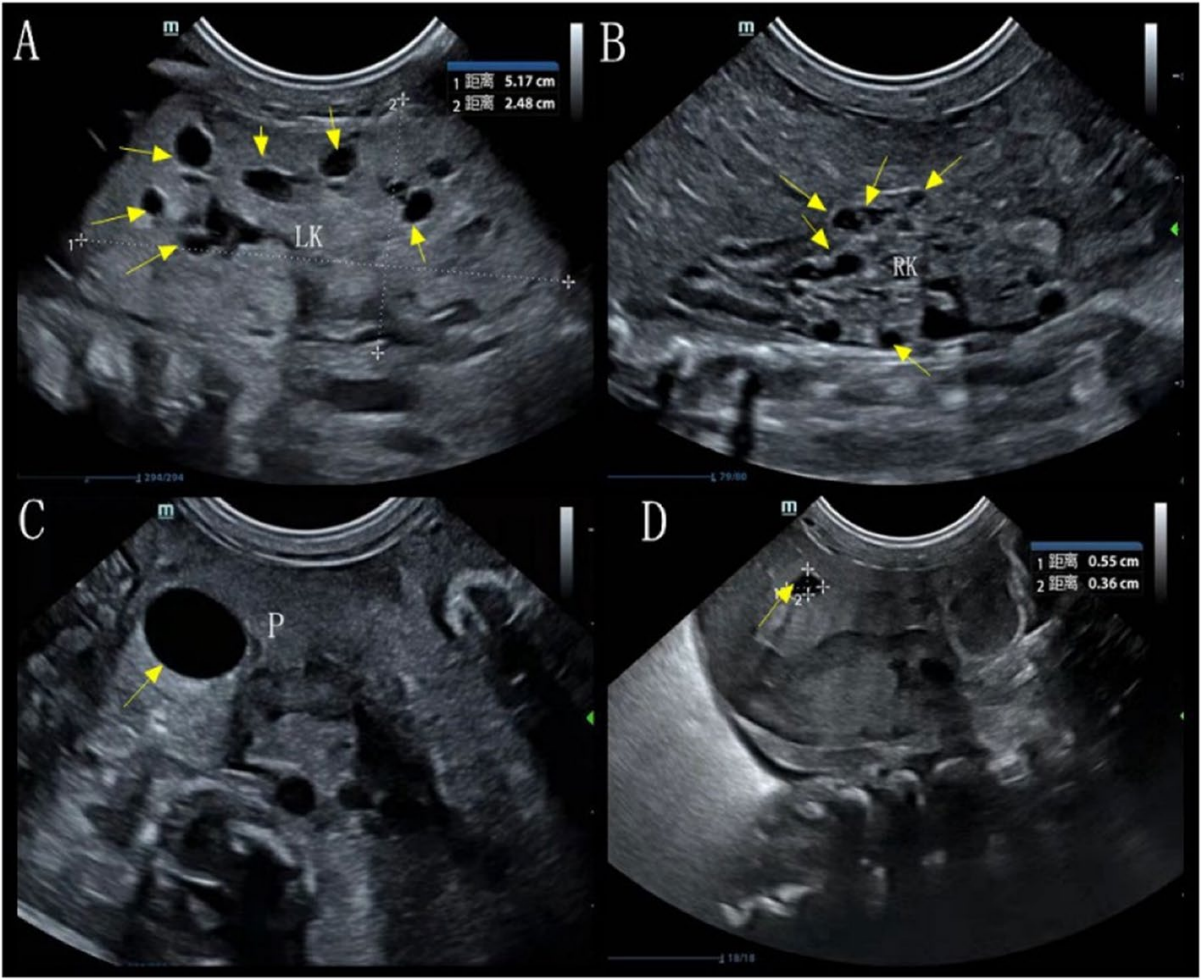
Ultrasound images of the upper abdomen showed the left kidney (arrows) multiple cysts (A), the right kidney (arrows) multiple cysts (B), (arrows) the head and tail of the pancreas contained two cysts (C and D)
